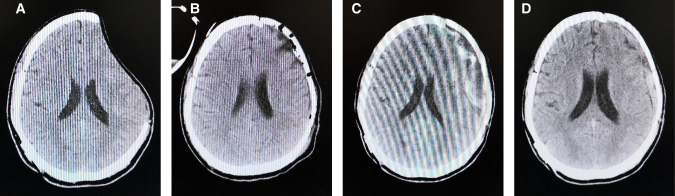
CT scan images before PEEK cranioplasty (A), right after the operation (B), day five post-operatively (C), and day ten post-operatively (D).
